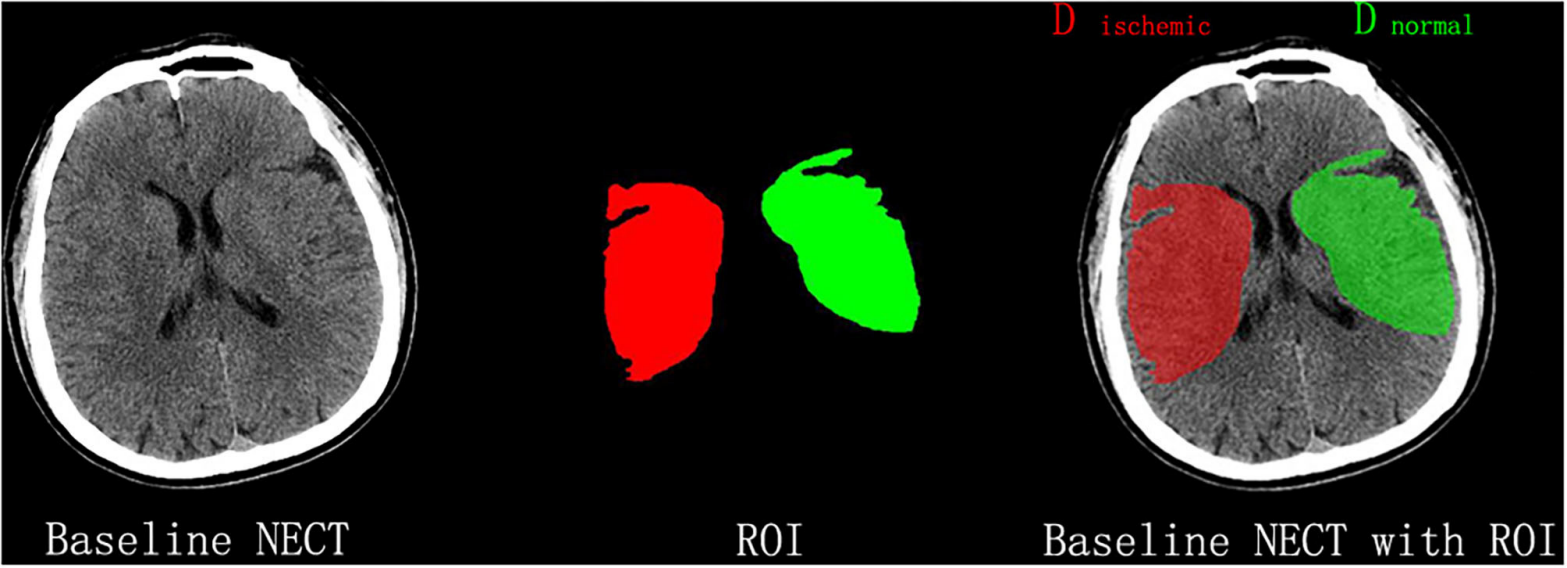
Measurement of NWU on admission nonenhanced computed tomography (NECT). According to the clinical manifestations, the infarct lesion occurred in the right middle cerebral artery territory, which was blindly outlined. The mean density of the territory (Dischemic) and a mirrored contralateral region of interest (Dnormal) was then measured to calculate NWU.
